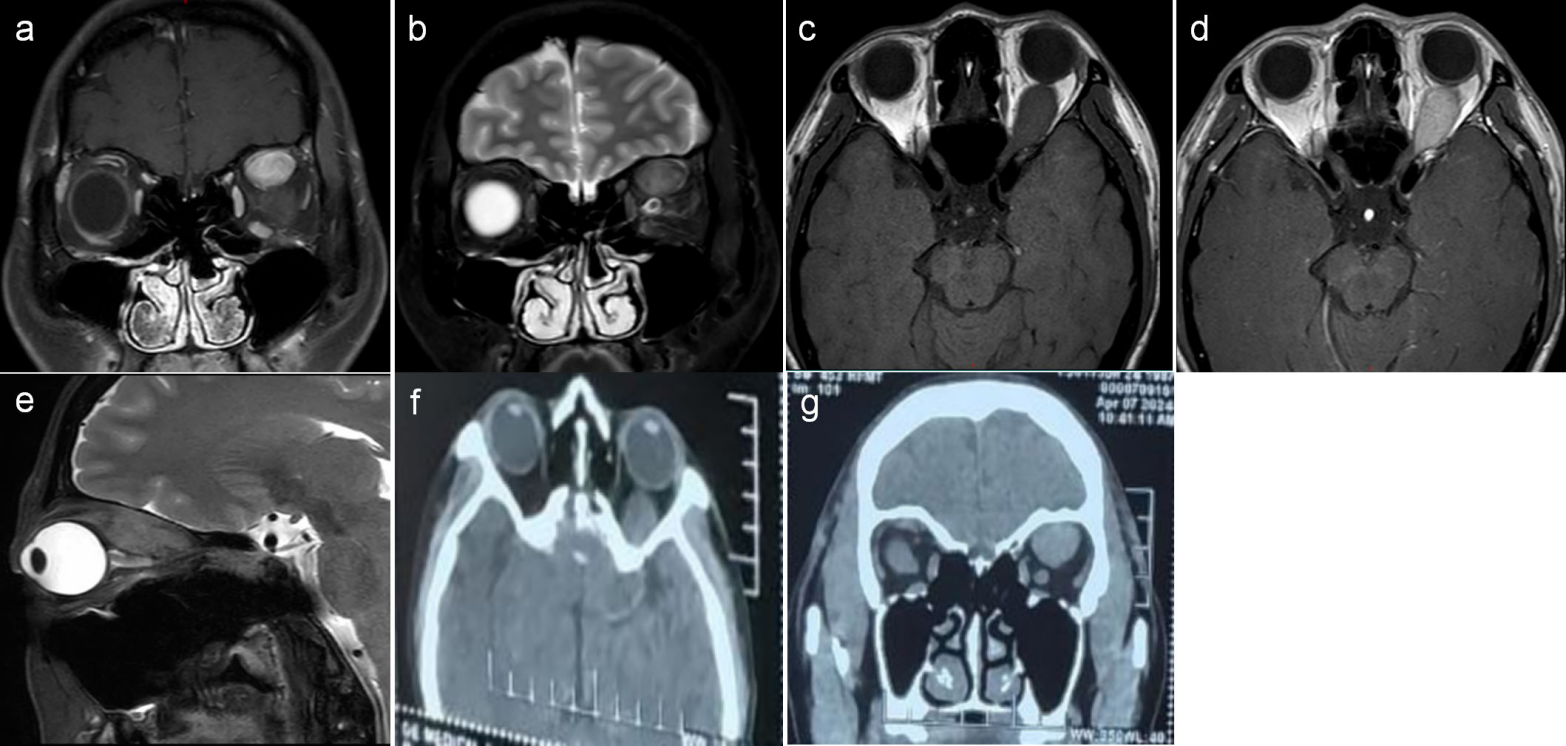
(A–E) Magnetic resonance imaging (MRI) showed a clear, oval mass in the left superior rectus muscle region, with intermediate T1 and slightly longer T2 signal intensity. The lesion was significantly enhanced on the scan, with small patchy weak enhancement areas visible inside. (F, G) Computed tomography (CT) scan showed a mass in the posterior cone of the left orbit, with an unclear boundary with the superior rectus muscle.
